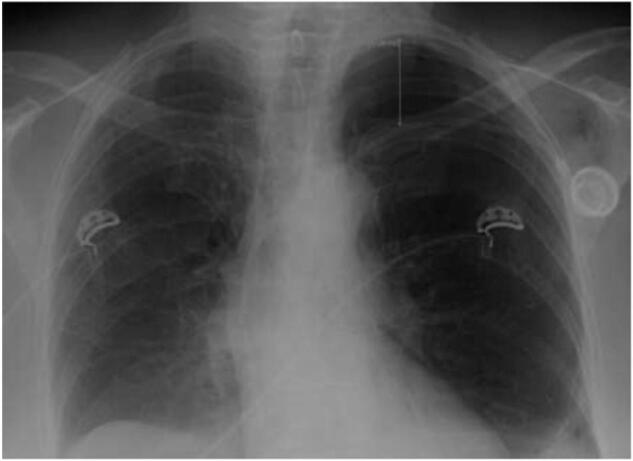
Chest X-ray of a patient with pneumothorax.
